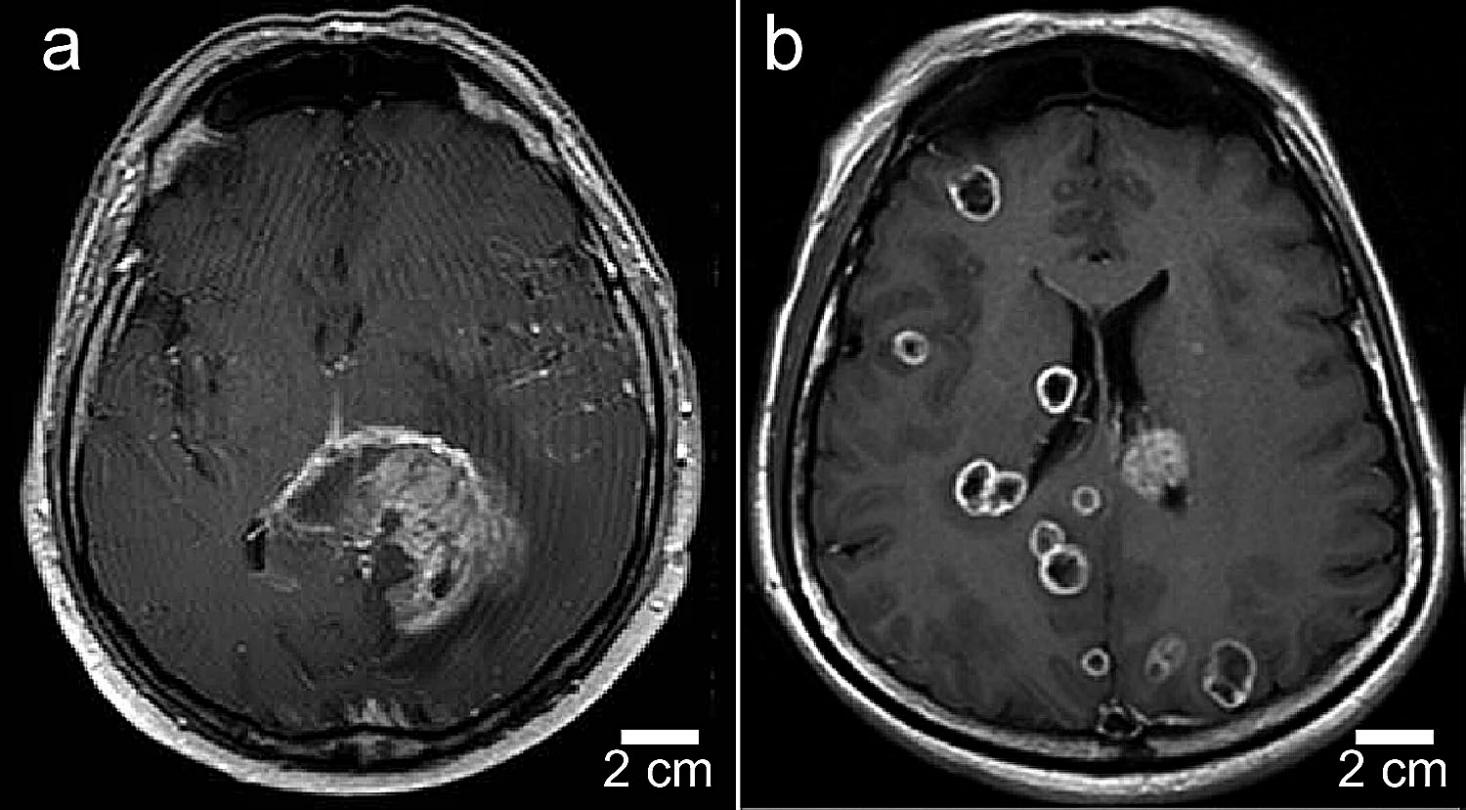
Human T1-MRI of glioblastoma multiform (a) and TNBC breast metastasis (b). Mets have rings of enhancement showing more active growth. GBM is more heterogeneous and diffuse with extensive edema and infiltrative tumor. Figures from references^28,29^, courtesy of John Wiley and Sons, Copyright 2017 by the American Society of Neuroimaging, and Taylor & Francis Ltd., www.tandofonline.com).
